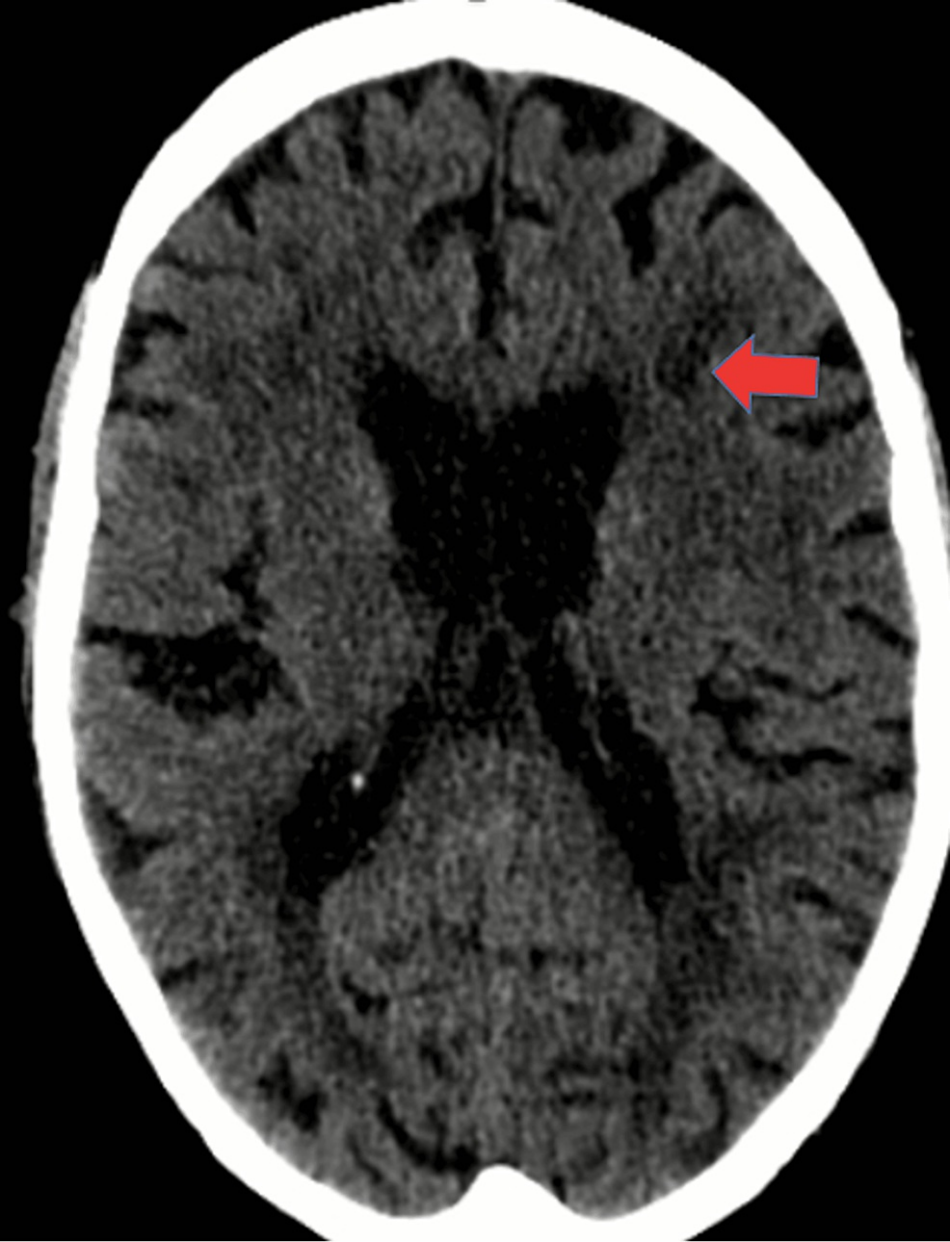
CT revealing the presence of cortico-subcortical insular and frontal hypodensity, indicating recent ischemic injury of the left MCA (indicated by a red arrow)
